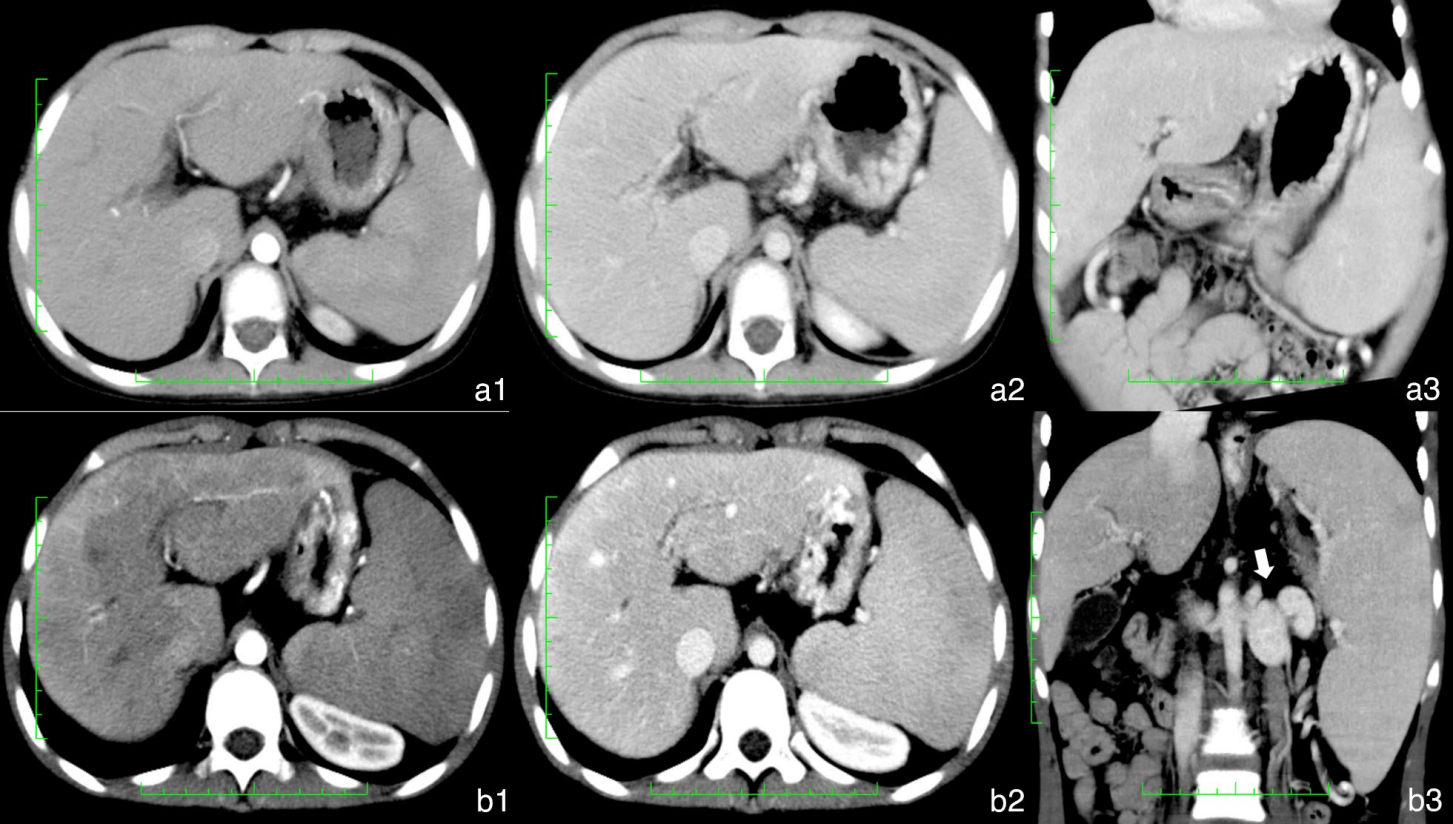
Male patient aged 6 years 4 months with a 3-year history of repeated hematemesis. a Complete loss of vascular landmarks of the Rex recess (type 4). The patient underwent the Warren procedure. b Computed tomography angiography after 56 months. Complete loss of vascular landmarks of the Rex recess. b3 The spleen–kidney vein anastomosis and the spleen were larger than before
